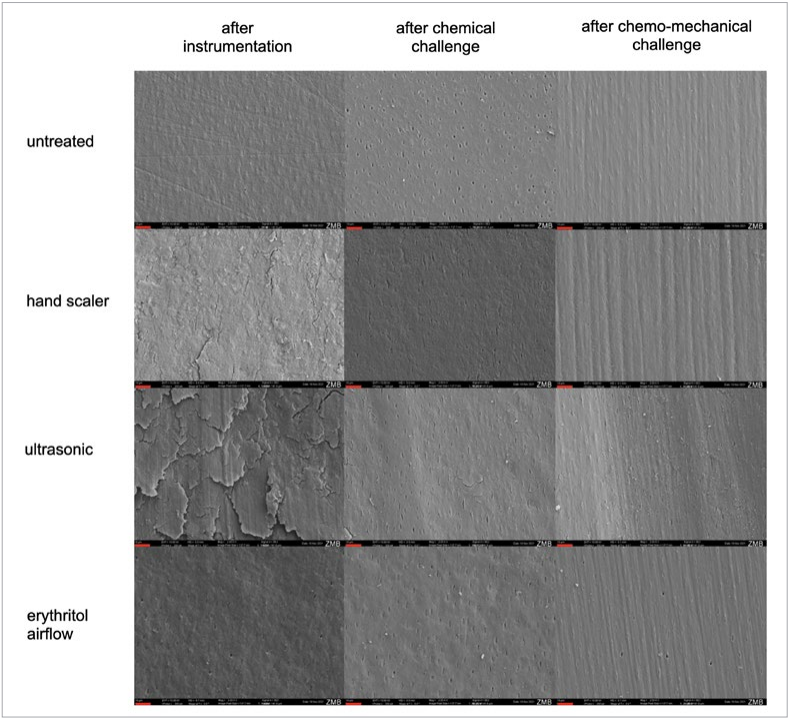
SEM images of dentin surfaces after instrumentation with the hand scaler, ultrasonic device, airflow, or left untreated, after chemical/chemomechanical challenge. The red line marks 10 µm.
